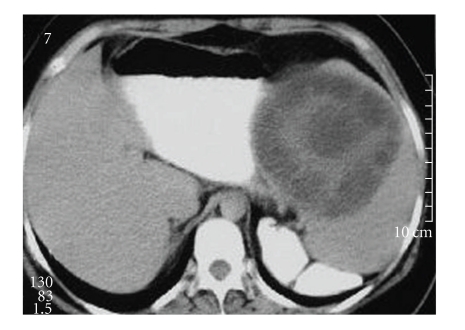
Axial CT with oral and IV contrast shows an unenhanced hypodense mass with well-defined borders in the anterior part of the spleen. Multiple round daughter cysts are seen peripherally within the lesion.
